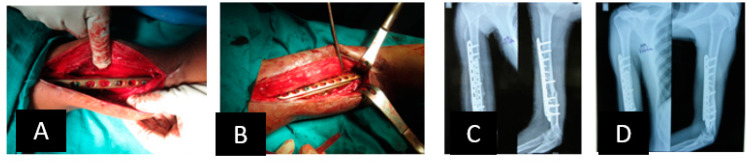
After six weeks, membrane formation was induced after removal of the cement spacer in stage two. (A,B) The defect was filled with a bone graft and an additional orthogonal plate for enhanced rotational stability. (C) Subsequent postoperative X-ray, AP and lateral views. (D) AP and lateral radiographs at the one-year follow-up showed successful healing.
